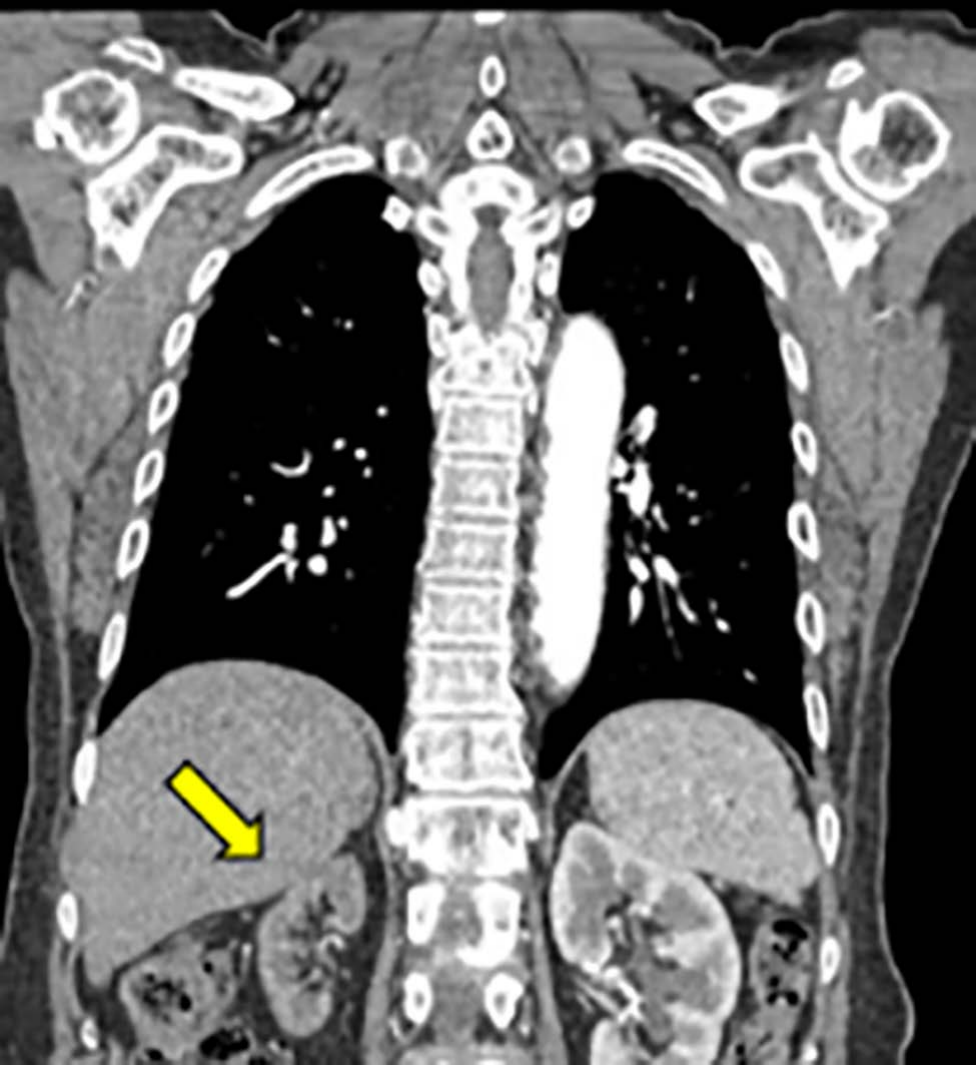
Right kidney atrophy (yellow arrow) was observed on computed tomography performed 2 months prior to the first percutaneous coronary intervention procedure.
